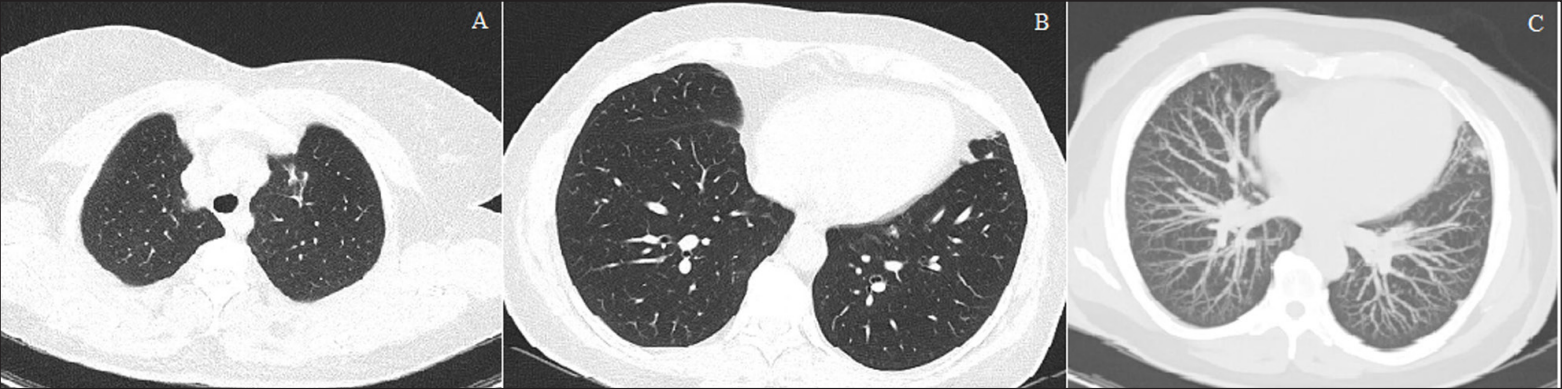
In control chest CT (45 days after disease onset), nodules in the upper lobes and GGO have completely disappeared, and a sequelae band is observed in the place of the nodule in the left anterior segment in Figure 1A. (A). Nodules and GGO have regressed almost entirely in the bilateral lower lobes. (B). MIP axial CT shows the disappearance of most nodules (C).
